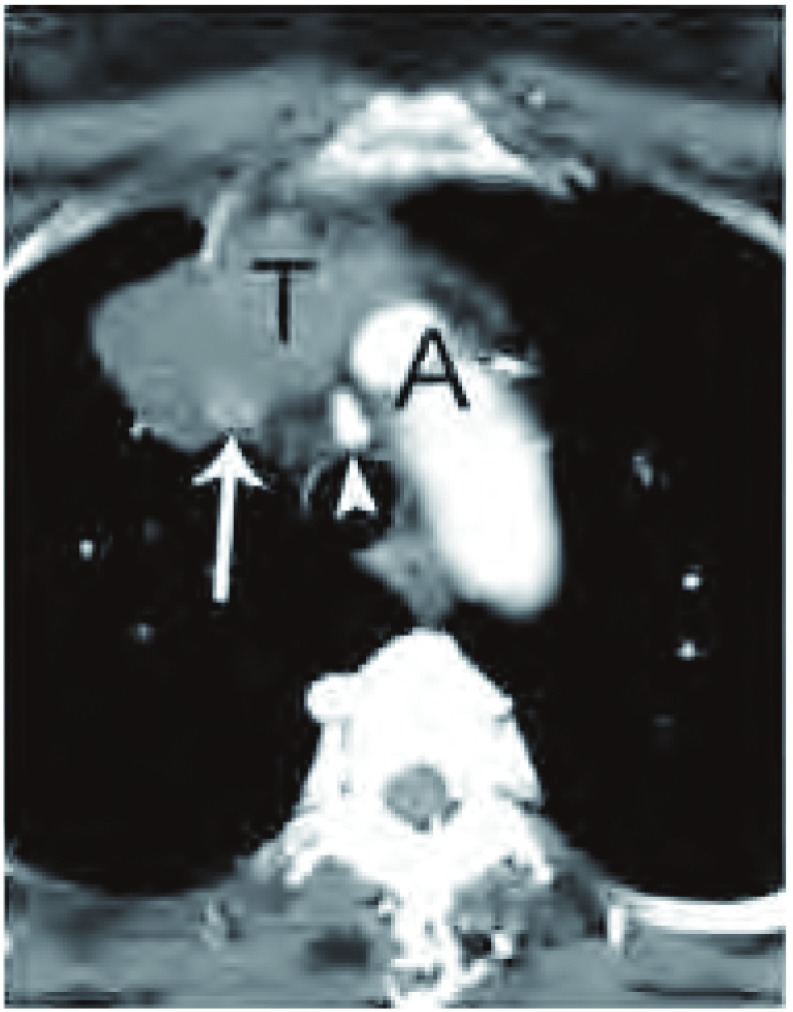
肿瘤侵犯血管。增强胸部CT（软组织窗）示肺动脉干（P）水平，胸腺瘤直接侵犯上腔静脉（长箭头所示）。注意，管腔内肿物软组织密度与原发瘤相似。 Vessel invasion. Contrast- enhanced chest computed tomography (CT) (soft tissue window) at the level of the pulmonary trunk (P) demonstrates a thymoma in the anterior mediastinum (arrowhead) with direct invasion (seen on a more superior slice) into the superior vena cava (white arrow). Note soft tissue attenuation of the intracaval mass similar to that of the primary tumor.
